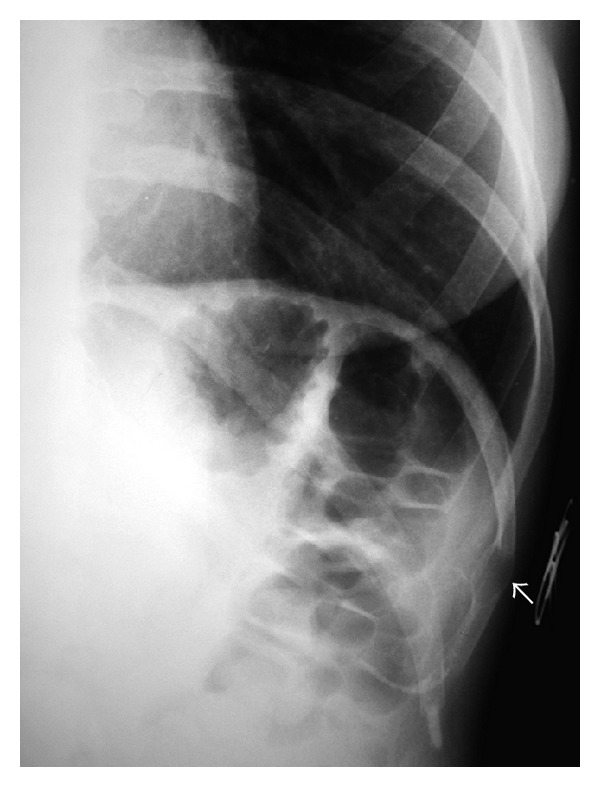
Conventional radiograph of the left lower ribs shows a well-circumscribed, ovoid lytic lesion (arrow) near the anterior aspect of the left tenth rib, causing thinning of the cortex and expansion of the bone.
